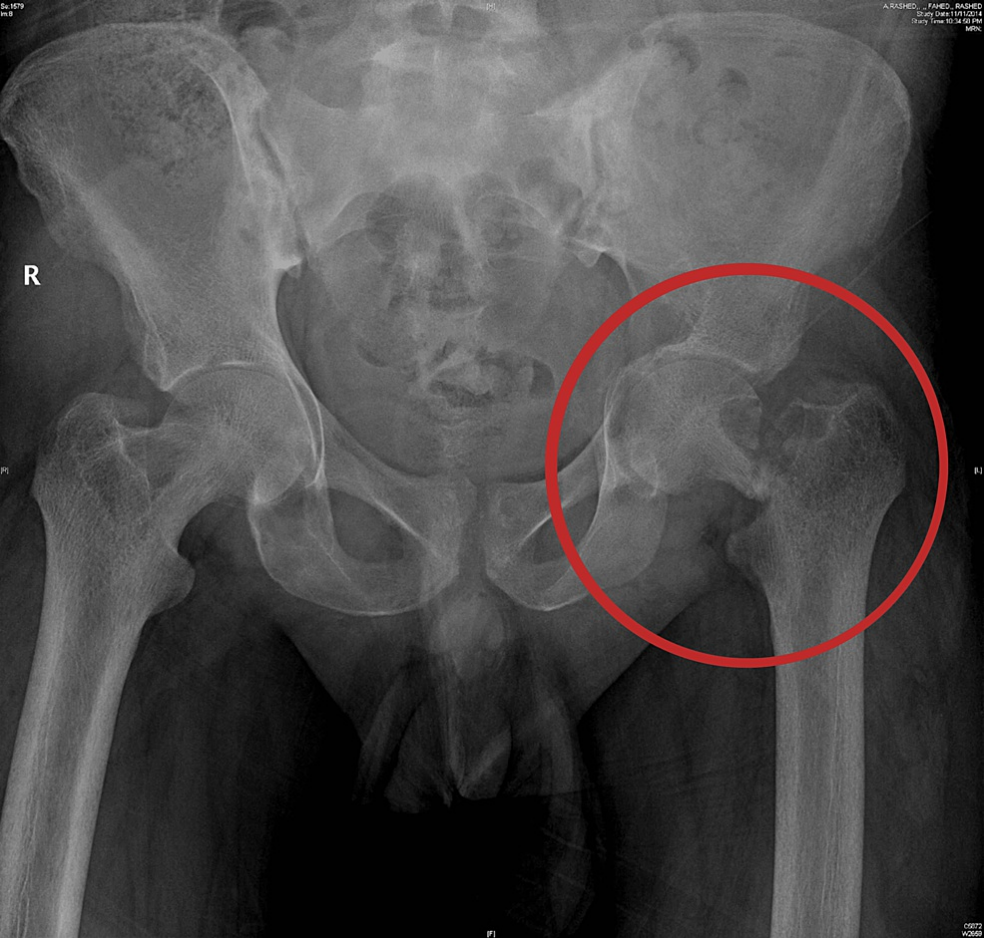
Plain radiograph of the pelvis: left neck of femur fracture, left protrusio acetabuli, and osteolytic lesion in the right neck of femur region
